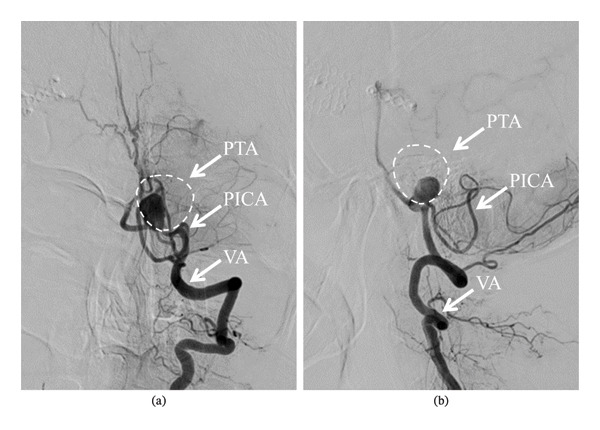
Left vertebral angiography performed before surgery (anteroposterior (a); lateral (b)) shows residual blood flow into the partially thrombosed aneurysm, suggesting that posterior inferior cerebellar artery (PICA) originates from the aneurysm dome. The dotted circle indicates the estimated outer diameter of the partially thrombosed aneurysm based on MRI measurements; PICA: posterior inferior cerebellar artery, PTA: partially thrombosed aneurysm, VA: vertebral artery.
